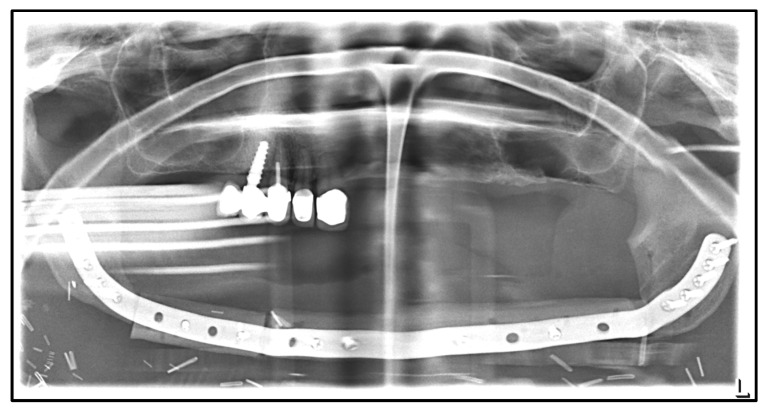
Dental X-ray after mandibular resection and primary bony reconstruction using a free fibular flap, April 2020.
